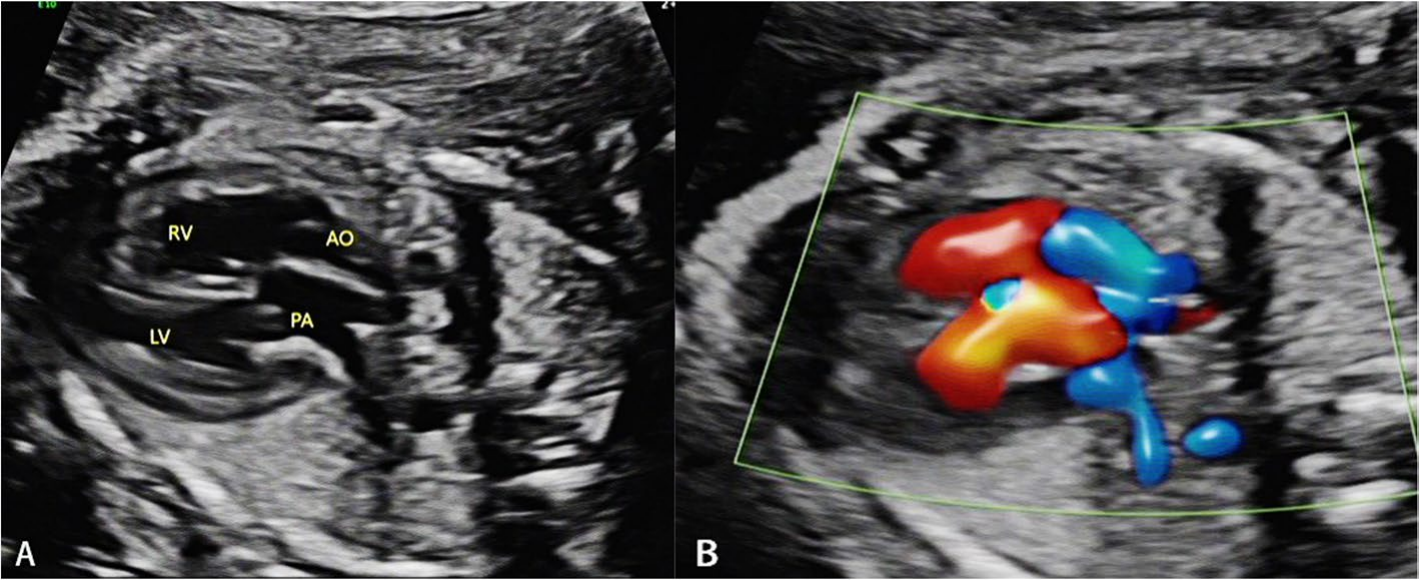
Inconsistent ventricle-vessel connections and the parallelism of the great arteries in two ventricular outflow tracts of TGA. A 2-dimensional mapping; B Doppler color flow mapping. RV, right ventricle; LV, left ventricle; AO, aorta; PA, pulmonary artery
